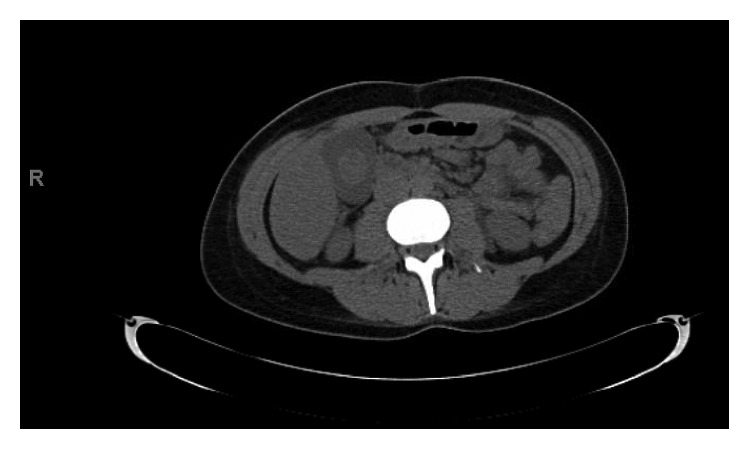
CT abdomen and pelvis without contrast demonstrating mild perihepatic ascites, diffuse low-attenuation of the liver likely due to active hepatocellular disease. Diffusely thickened gallbladder wall as demonstrated on abdominal ultrasound. Additional CT findings include a moderate amount of free fluid in the pelvis and a small right-sided pleural effusion. No obstructing renal calculus identified.
